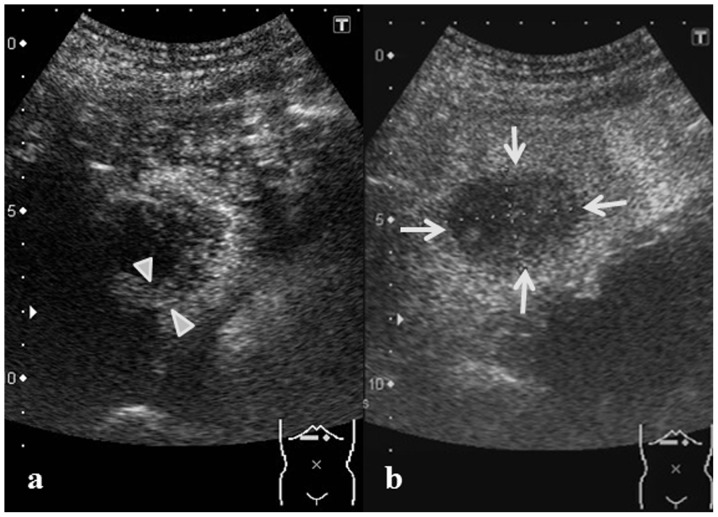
Abdominal contrast-enhanced ultrasonography at the time of admission. (a) A contrast enhancement effect of ~5 mm was indicated at the tumor border in the vascular phase in the tumor in the S2 liver segment (arrowheads). (b) A defect in the entire tumor was observed in the post-vascular phase (arrows).
